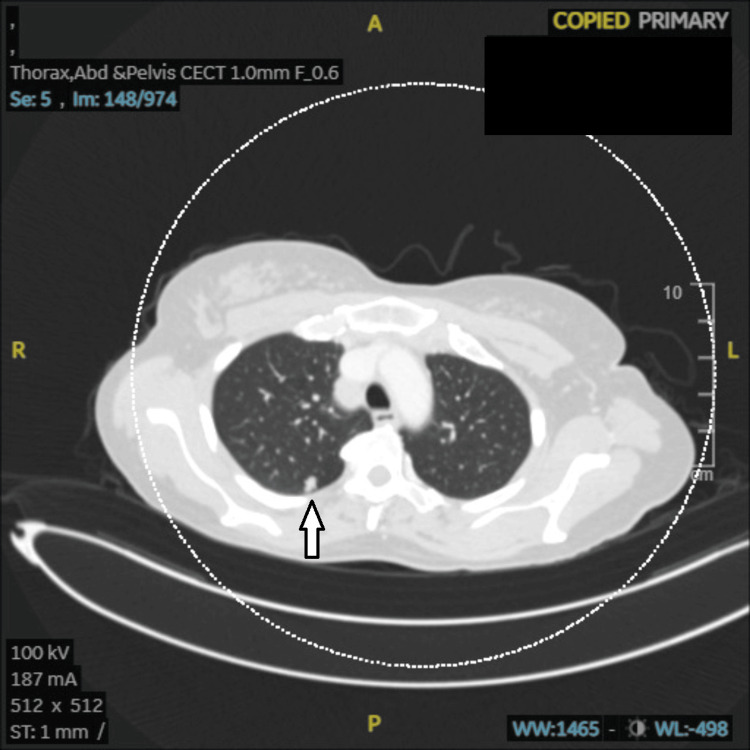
Case 1: CECT (chest) showing right upper lobe posterior segment, well-defined non-calcified nodule with lobular end margins (8.5 × 7 mm) likely benign (arrow). CECT: contrast-enhanced computed tomography, A: anterior, R: right, L: left, P: posterior, ST: soft tissue, WW: window width, WL: window level.
